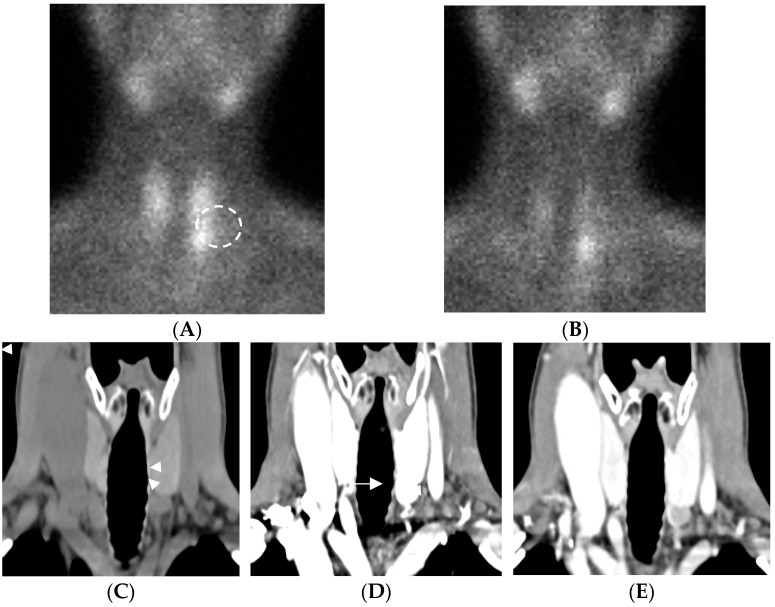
Left inferior parathyroid adenoma in a 50-year-old woman. (A) Scintigraphy with 99mTc-sestamibi: a nodular uptake (dashed circle) is observed on the left side, slightly more intense than the uptake in the adjacent superior portion of the thyroid lobe. (B) On the delayed scan, this focal lesion shows a clearly delayed washout compared to the surrounding thyroid tissue. 4D-CT coronal reformatted images: (C) hypodense nodule (arrowheads) on plane image with respect to thyroid gland, (D) enhancing on arterial phase, and (E) hypodense on venous phase with respect to thyroid gland. “Polar vessel sign” (arrow) is clearly visible. The 4D-CT scan shows no thyroid tissue around the parathyroid gland (no intrathyroidal parathyroid).
